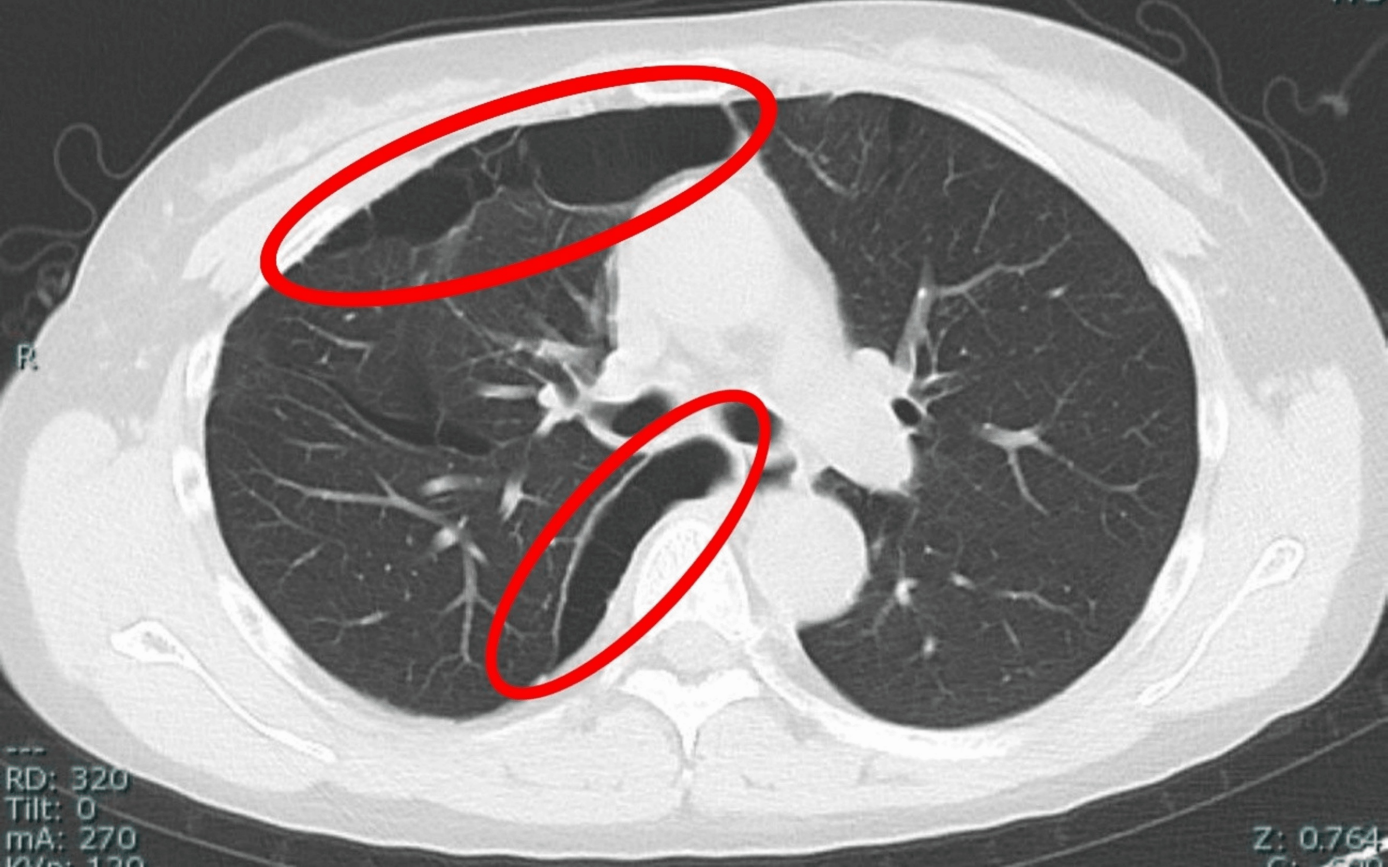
Preoperative chest CT (axial) showing multiple pulmonary cysts. Multiple pulmonary cysts are observed, predominantly in the right middle and lower lung fields, and along the mediastinal side (red ovals). CT, Computed Tomography
